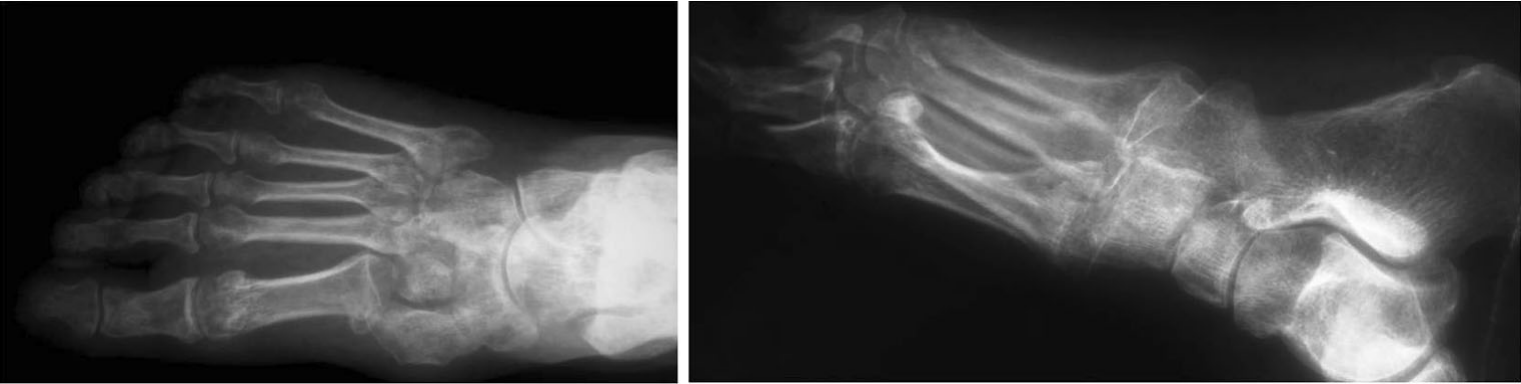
AP and lateral radiograph of involved foot on presentation
